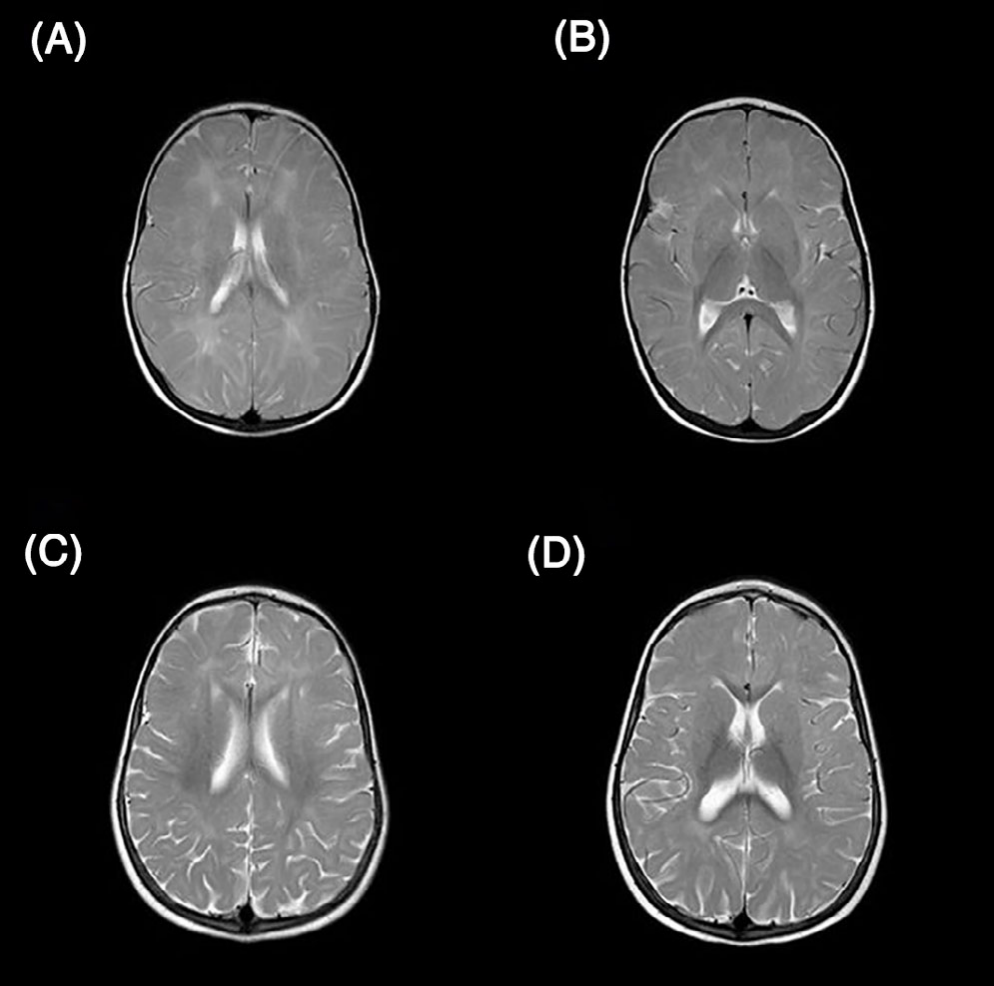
T2 brain MRI of sibling #1 showed (Α and Β) delay in myelination of subcortical and periventricular white matter at the age of 19 months with normal CNS structure, (C and D) persistence of the axonal damage at the age of 3 years after 6 months of folinic acid administration, accompanied by clinical improvement
